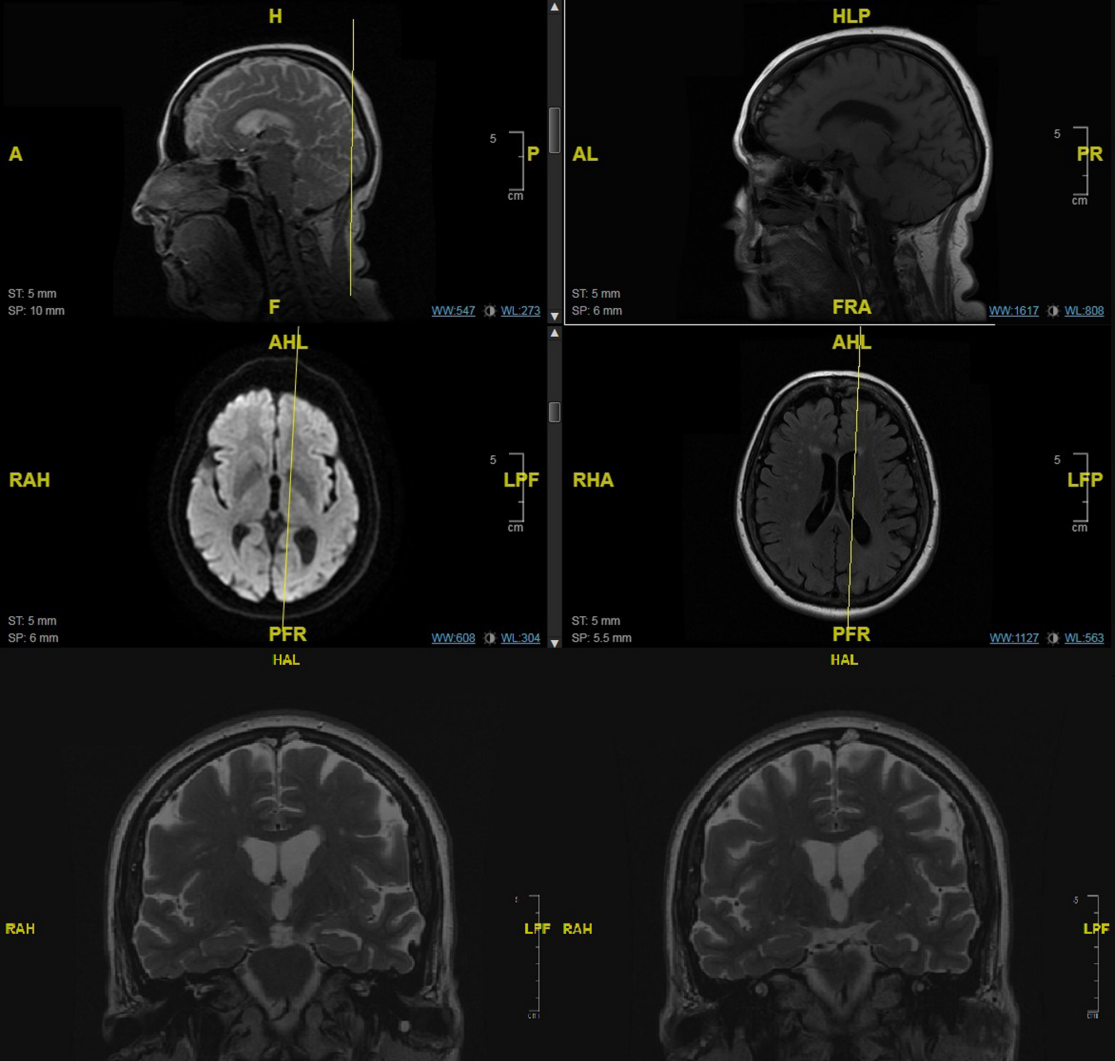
Brain MRI of the patient. The imaging was obtained at *T*
